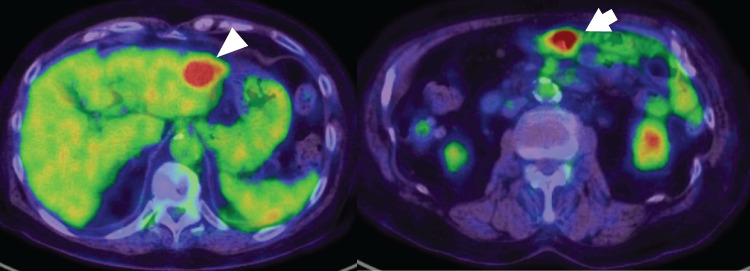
PET-CT image obtained at the initial visit. Fluorodeoxyglucose uptake is observed in the lateral segment of the liver (arrowhead) and transverse colon (arrow).
